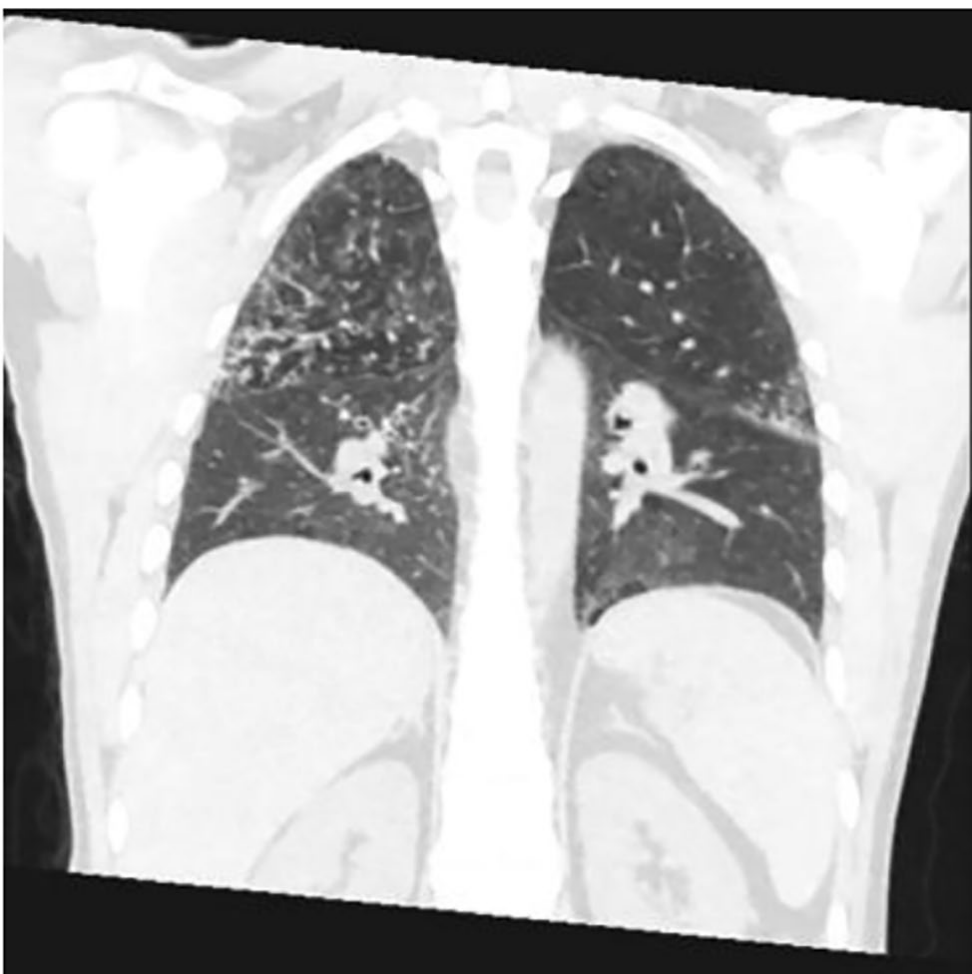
Coronal CT image shows bilateral peri-broncho-vascular nodules and ground glass opacities
